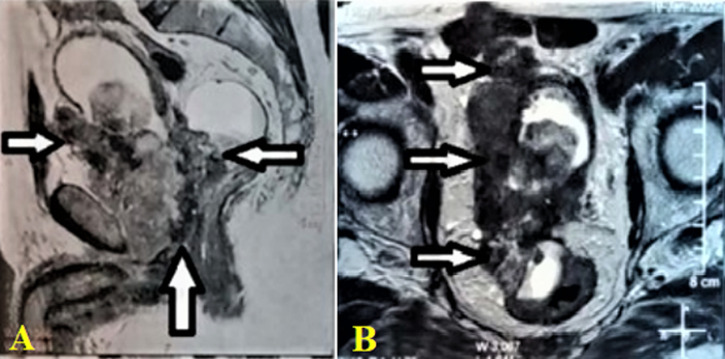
magnetic resonance imaging of the pelvis showing prostatic lesions (arrows) with expansion towards the two seminal vesicles (A); the lower and right lateral wall of the bladder, the rectal wall and the levator ani muscles, the rectal wall, the peri-prostatic bladder fat (B)
